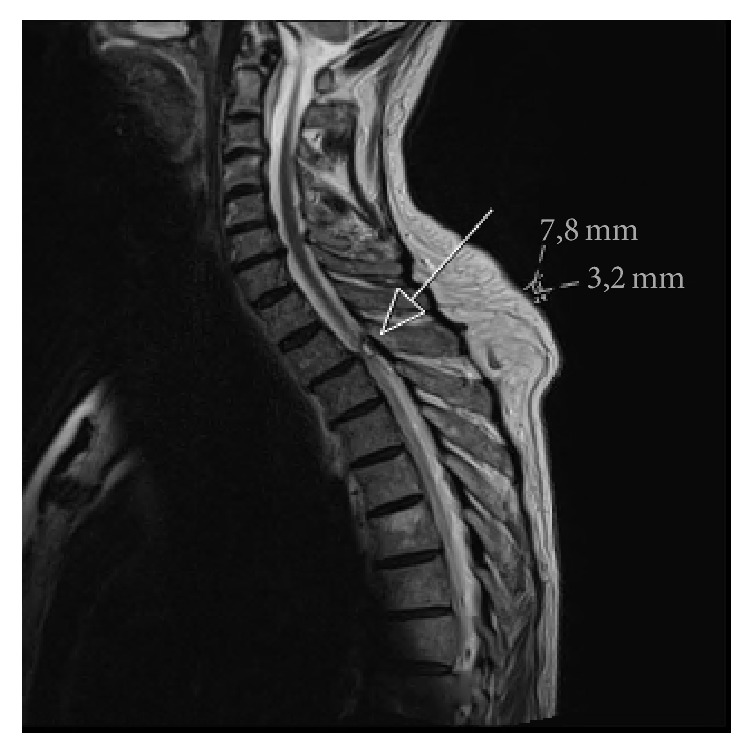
MRI of the upper spinal column T2-weighted sequence, sagittal plane. White arrow showing a 7,8 mm by 3,2 mm cyst at the Th2-3 level on the right side.
